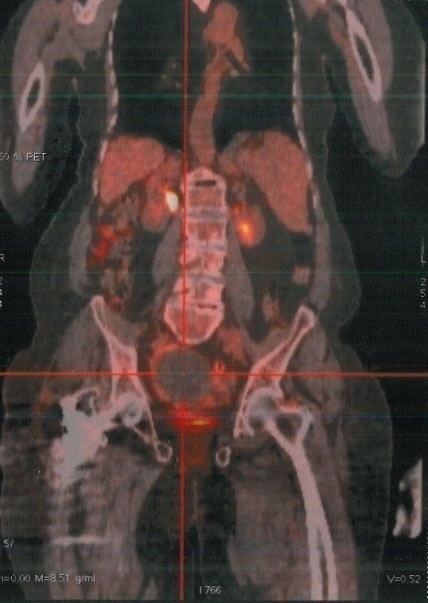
Positron emission tomography scans with FDG showing an intense hypermetabolisme in the pelvic (SUV max: 3.3) with no uptake in the liver
